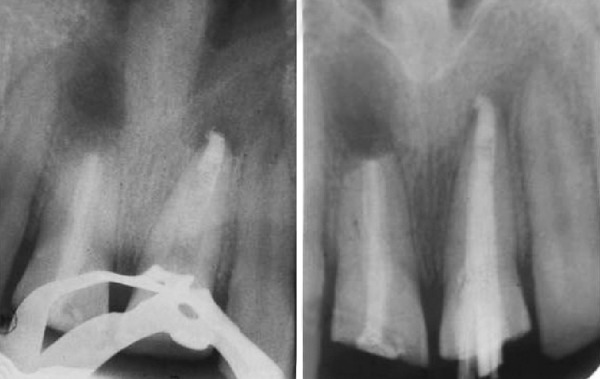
Periapical radiograph revealing apexification with MTA and endodontic treatment of 21
